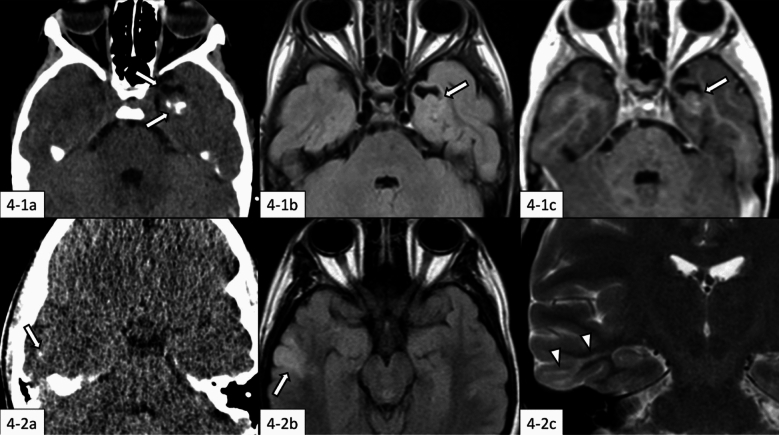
Polymorphous low-grade neuroepithelial tumor of the young. 4-1 (7-year-old girl): There is a solid and cystic tumor mass involving the cortical and subcortical regions in the left temporal lobe (4-1a–c, arrows). The tumor mass shows solid calcification on non-contrast computed tomography (CT) (4-1a), isointensity to the cortex on fluid-attenuated inversion recovery (FLAIR) imaging (4-1b), and scarce enhancement on contrast-enhanced T1-weighted imaging (4-1c). The same case with different images has been evaluated in our previous study [2]. 4-2 (17-year-old girl): There is a tumor mass involving the cortical and subcortical regions in the right temporal lobe (4-2a, 4-2b, arrows). The tumor mass shows punctate calcification on non-contrast CT (4-2a), hyperintensity on FLAIR imaging (4-2b) with hyperintense “transmantle-like sign” extending from the cortex to the ventricle on T2-weighted coronal imaging (4-2c, arrowheads). The same case with different images has been evaluated in our previous study [47]
